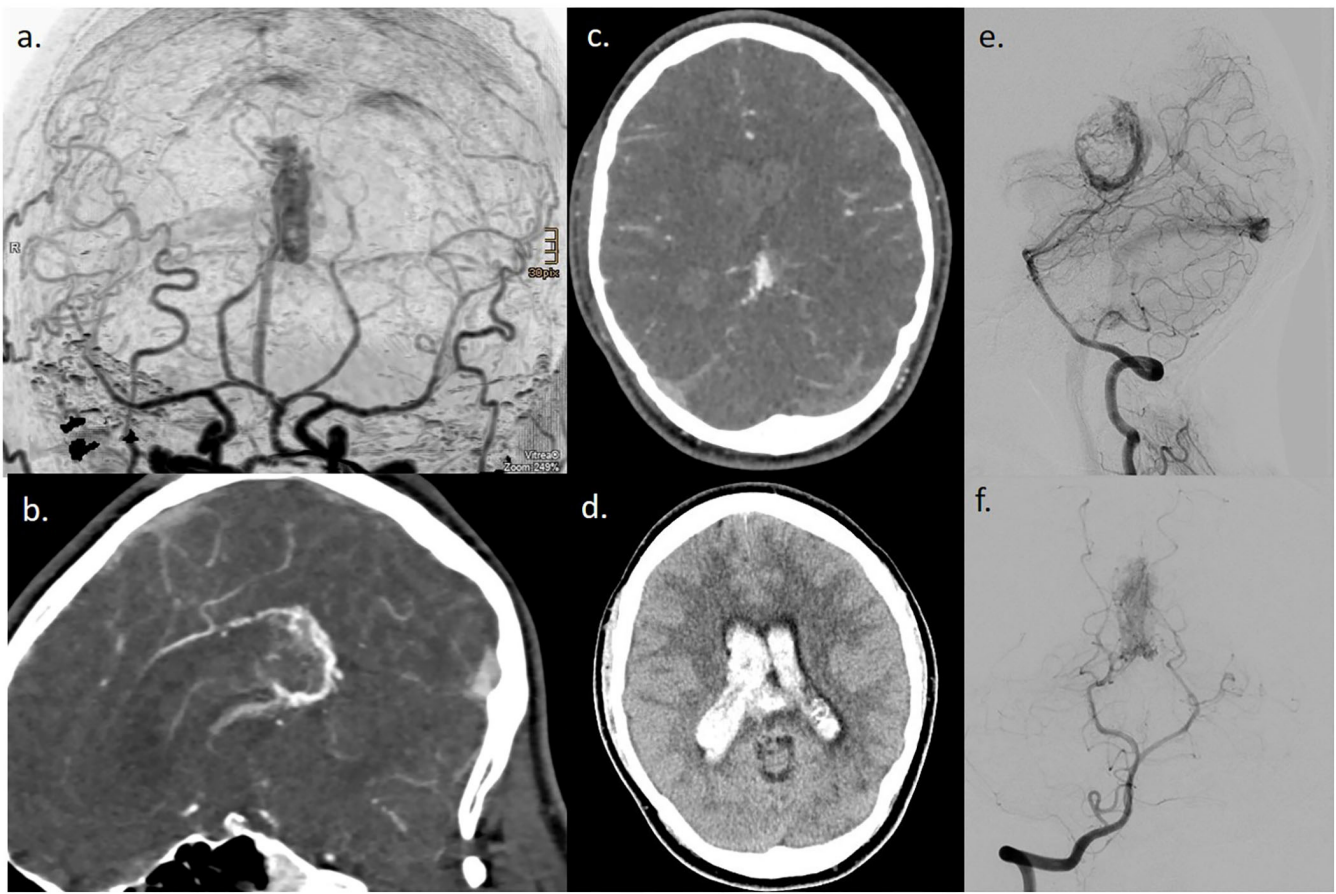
A case of a 28-year-old man with lobar ICH, and CT DSA images revealed no arterial hypertension. (a–d) CT/CTA images; (e,f) DSA images of an AVM at the splenium with hemorrhage.
